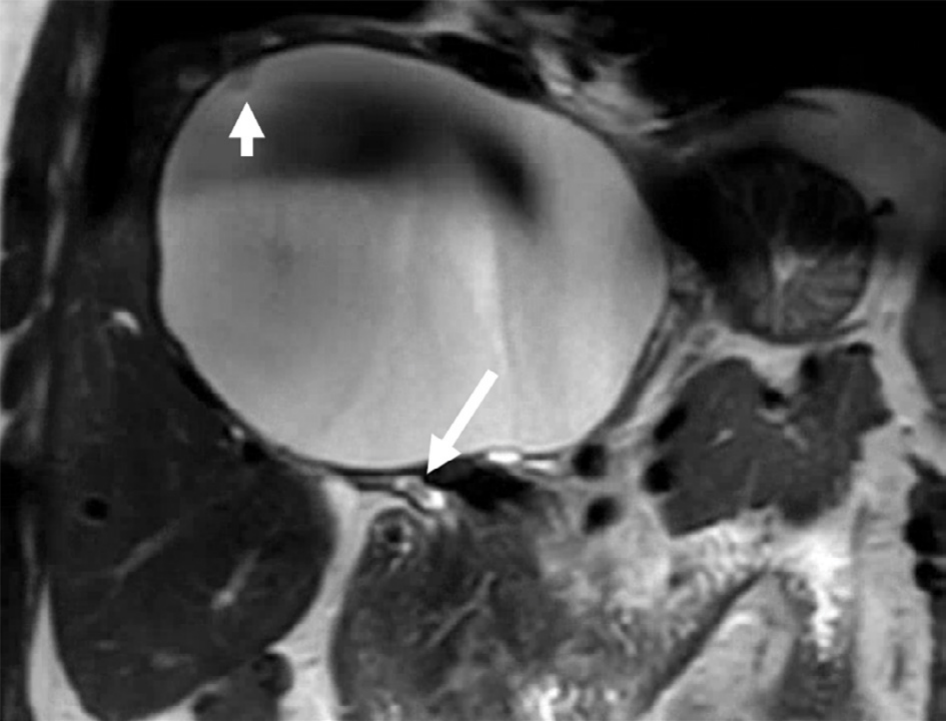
Coronal T2-weighted MR image shows a large cystic hepatic lesion with a small papillary projection (short arrow), peri-lesional intrahepatic bile duct dilatation, and a small filling defect in the extrahepatic bile duct (long arrow).
